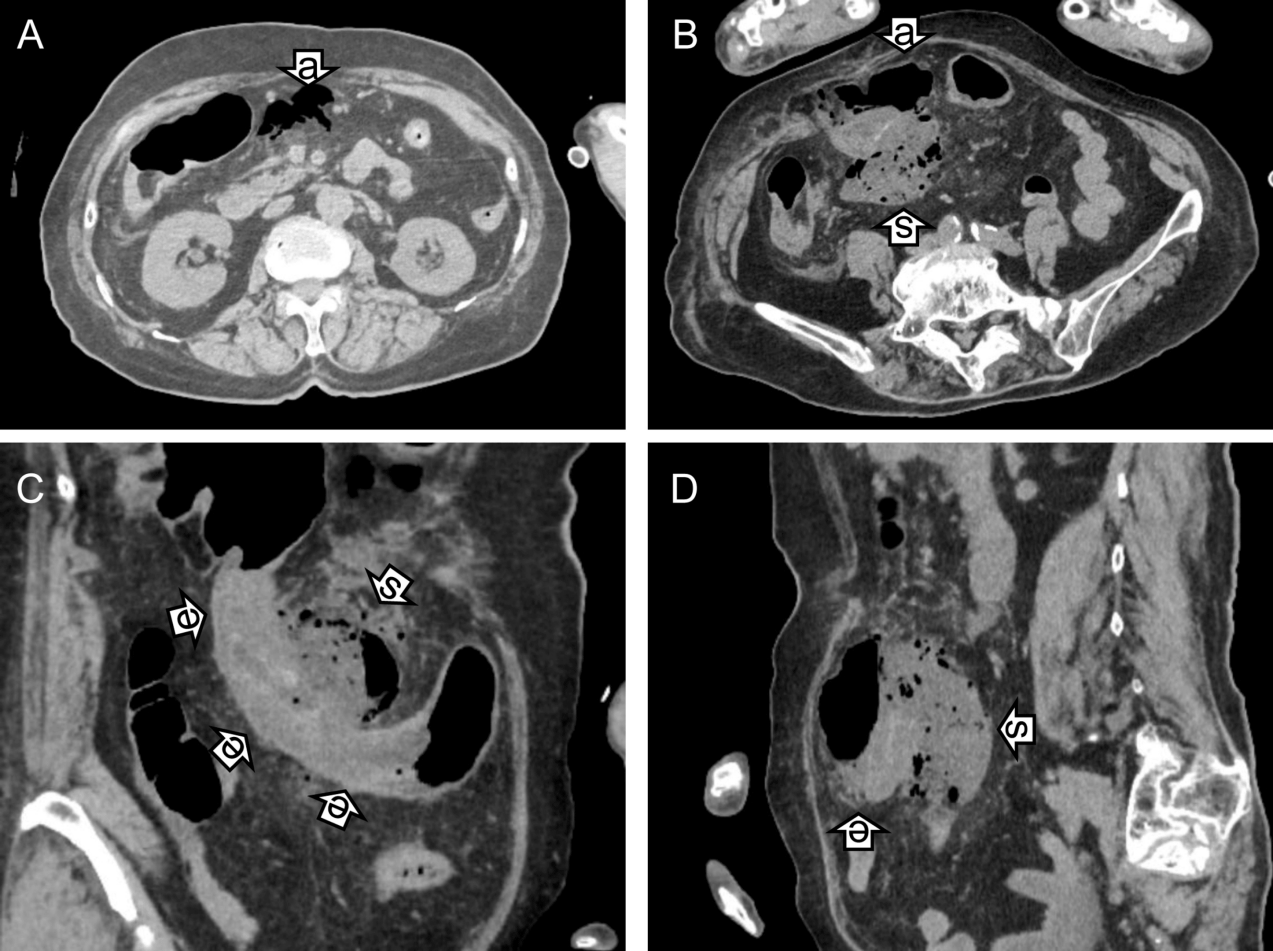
Computed tomography findings on day 2. On day 2, free air (arrows “a”, panels A and B), extraluminal feces (arrows “s”, panels B–D), and edematous transverse colon (arrows “e”, panels C and D) were evident on axial and coronal CT images.
